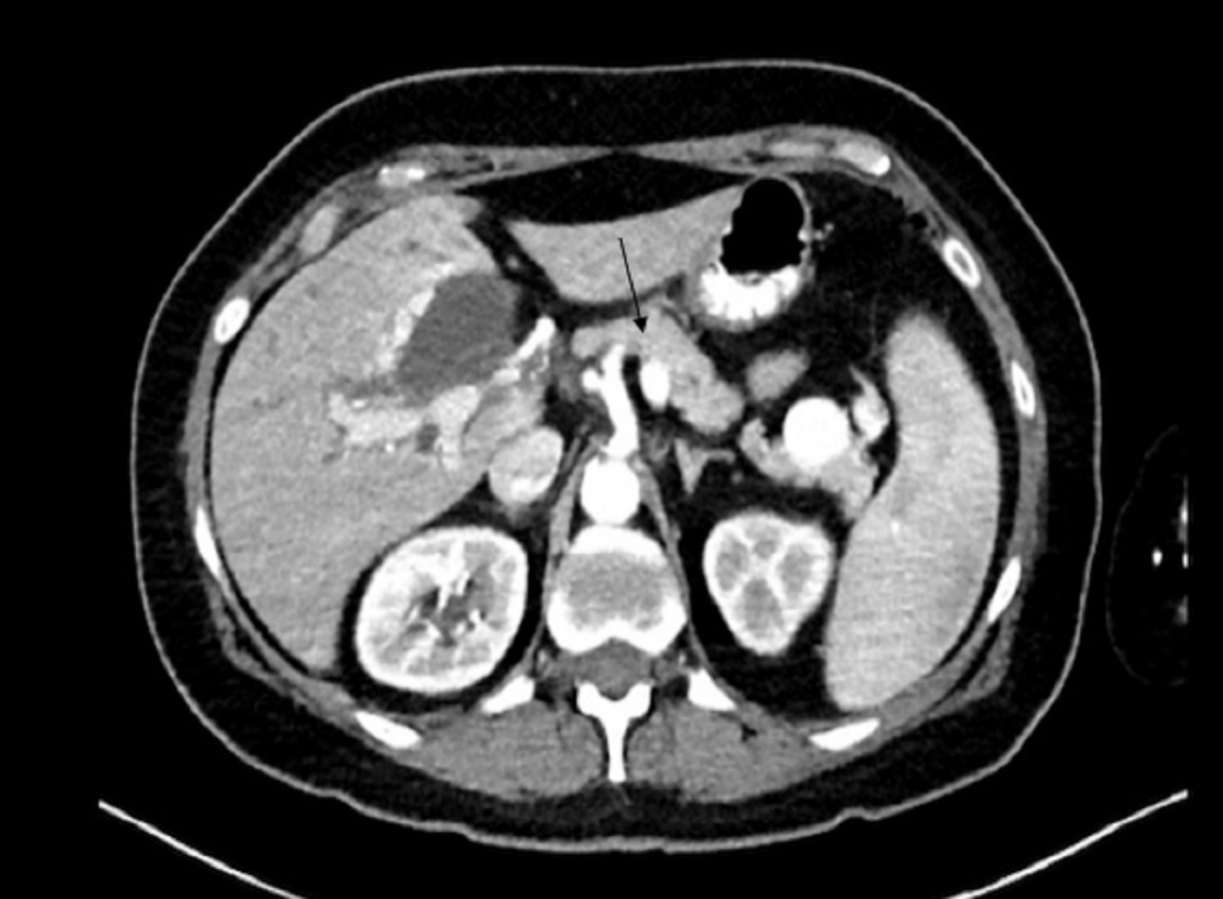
Computed tomography of abdomen with contrast suggestive of portal vein thrombosis, with arrow showing a markedly dilated splenic vein
